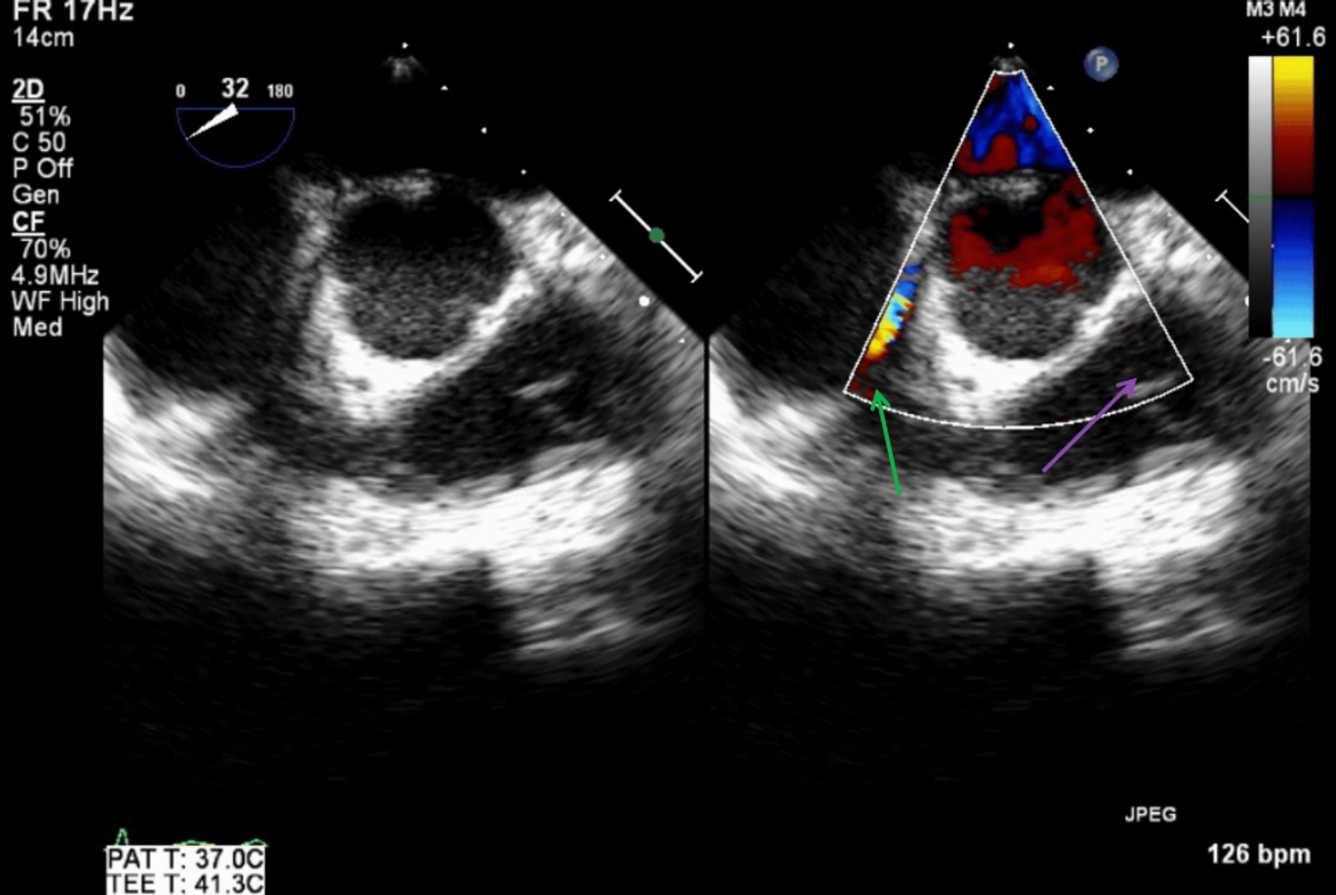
TEE mid-esophageal short-axis aortic valve view with and without color Doppler during diastole. The green arrow shows no findings of tricuspid valve regurgitation during diastole. The purple arrow shows no findings of pulmonic valve regurgitation during diastole. TEE: transesophageal echocardiography
